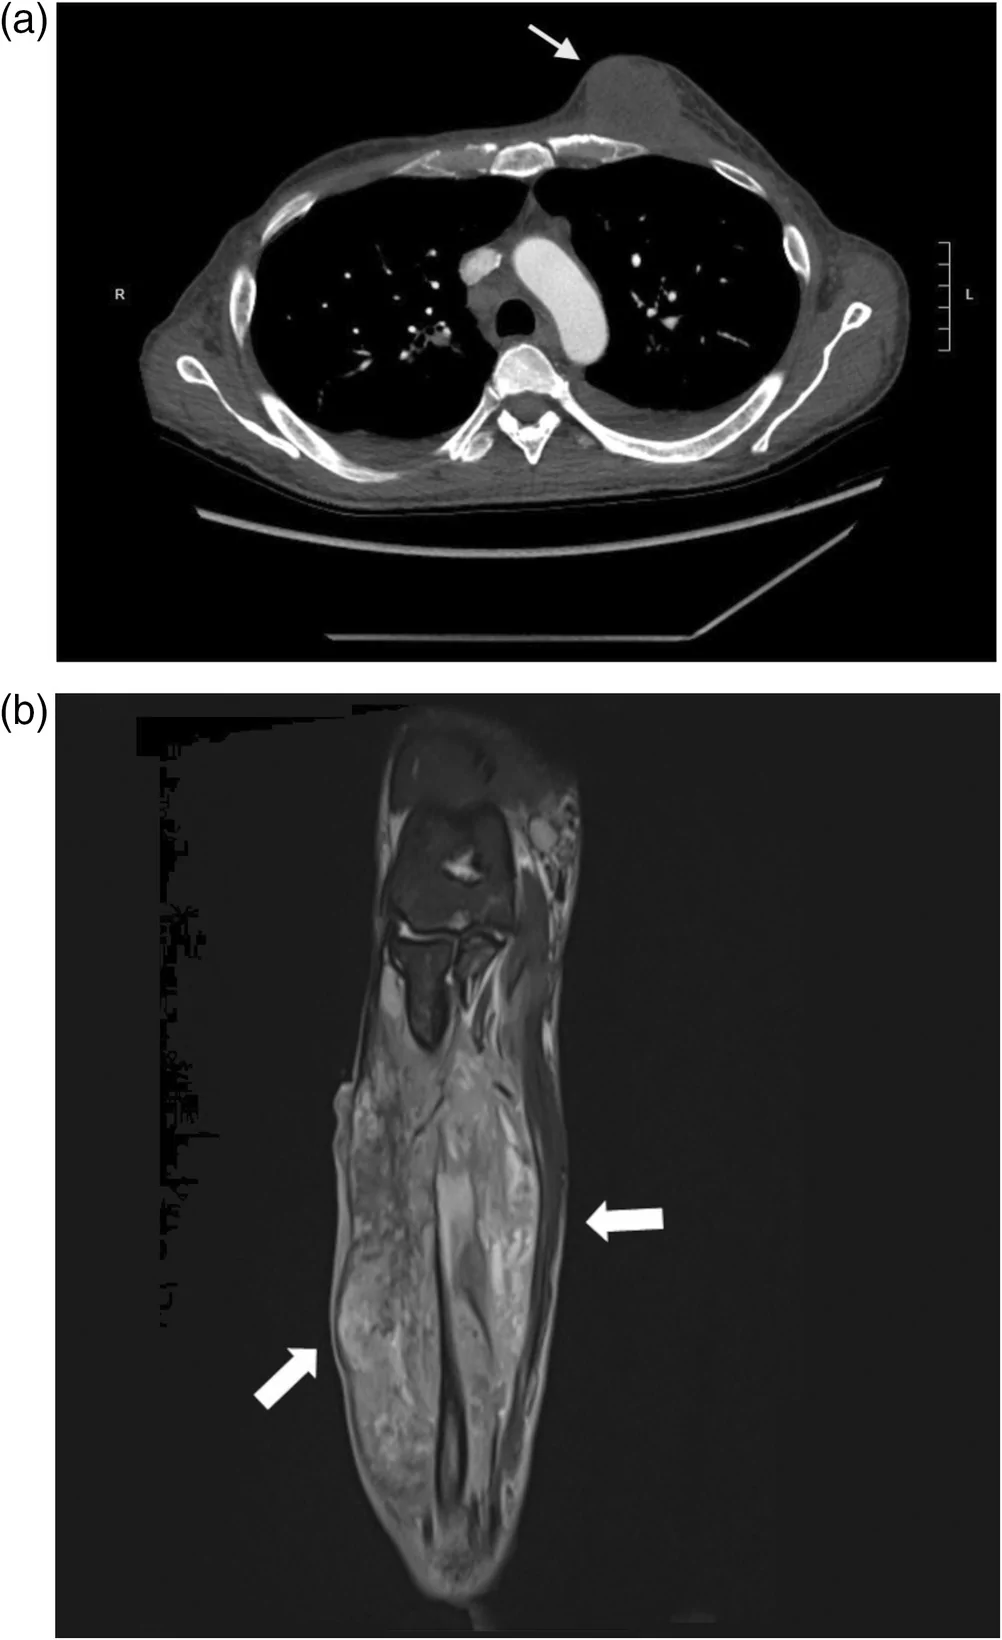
Radiological images of the right forearm and chest wall mass. (a) The CT showed a 2.8 cm heterogeneously enhancing soft tissue nodule (arrow) in the left medial upper chest wall, involving the subcutaneous layer and pectoralis muscle. (b) The MRI showed extensive heterogeneous T2 hyperintense enhancing infiltrative intramuscular mass involving both flexor and extensor compartments of the forearm (arrow).
